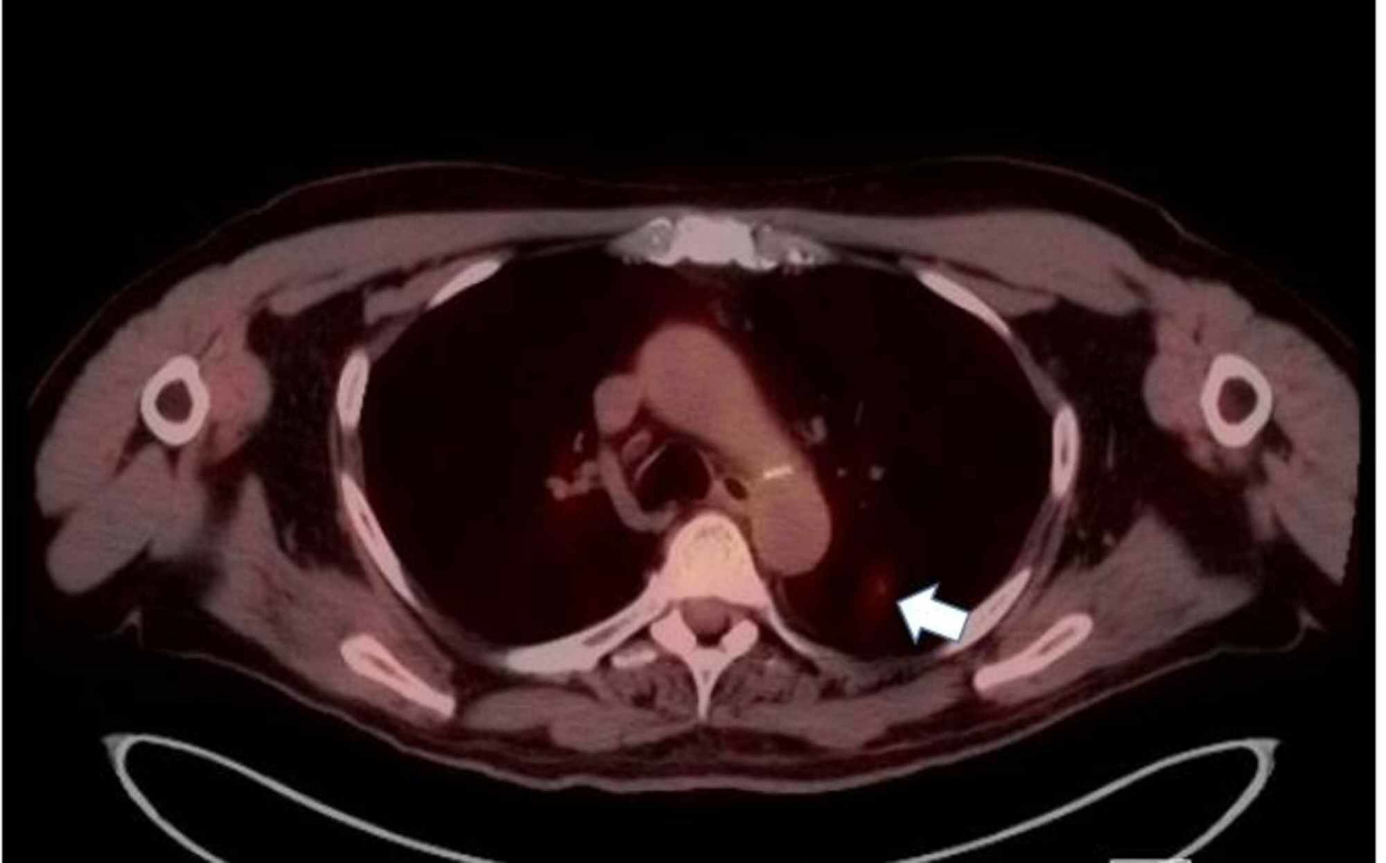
CT/PET scan of the lung in an axial view shows abnormal uptake of the 10-mm nodule in the left lower lobe of the lung (white arrow) CT: computed tomography; PET: positron emission tomography
